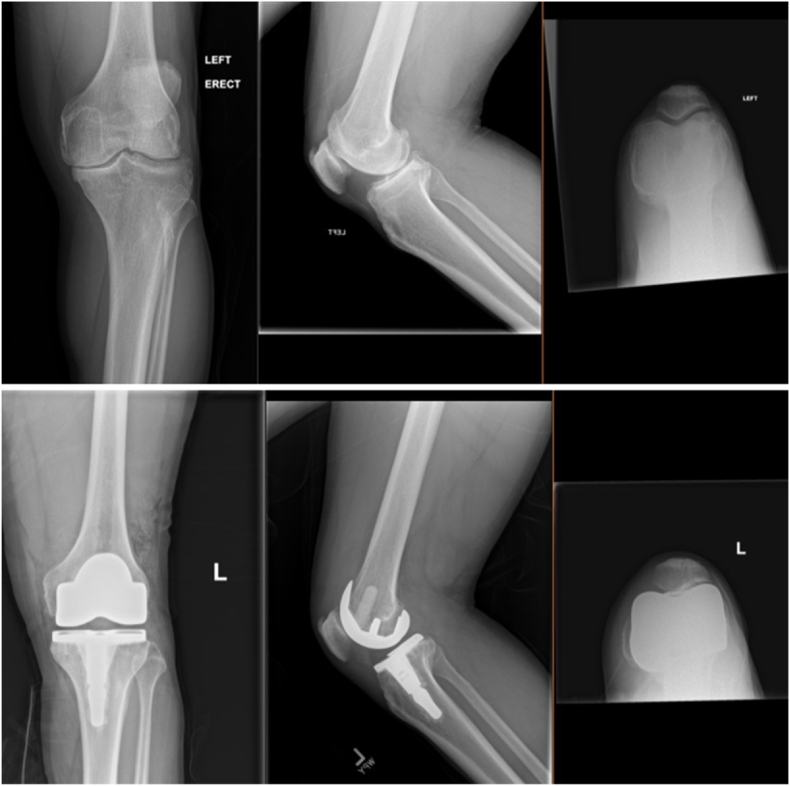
Pre- & post-operative radiographs demonstrating a medial pivot implant.
